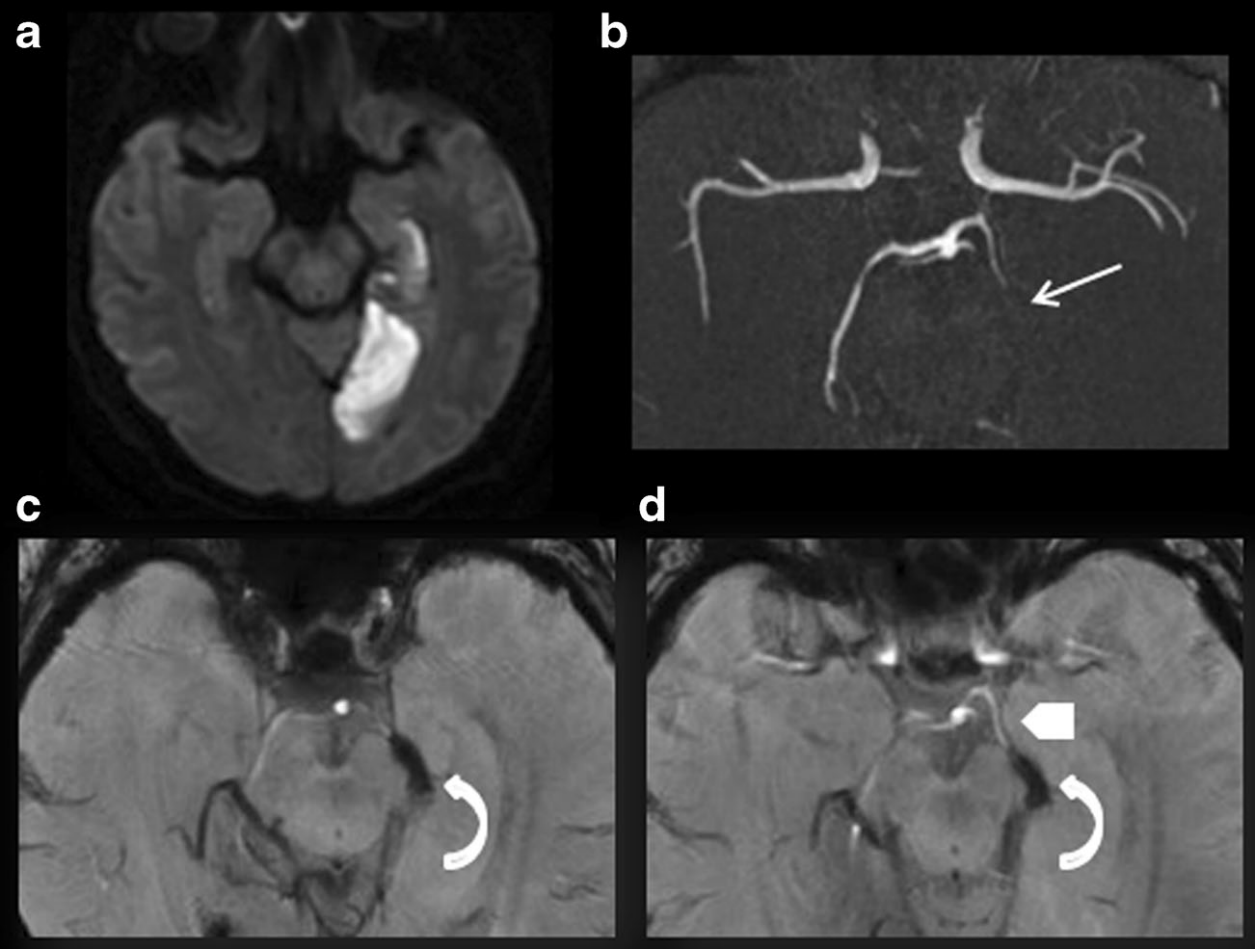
A 72-year-old patient with right homonymous lateral hemianopsia. DWI (a), 3D TOF (b), minimum intensity projection (c), and multiplanar reconstruction (d) of the SWAN sequence in the axial plane. a Acute ischaemic lesion in the territory of the left posterior cerebral artery. b No visualisation of the left P2 (*white arrow*). c and d Susceptibility vessel sign in P2 (*curved arrow*). d TOF effect of the SWAN sequence identifies the susceptibility vessel sign associated with the thrombus and the upstream arterial segment (arrowhead)
